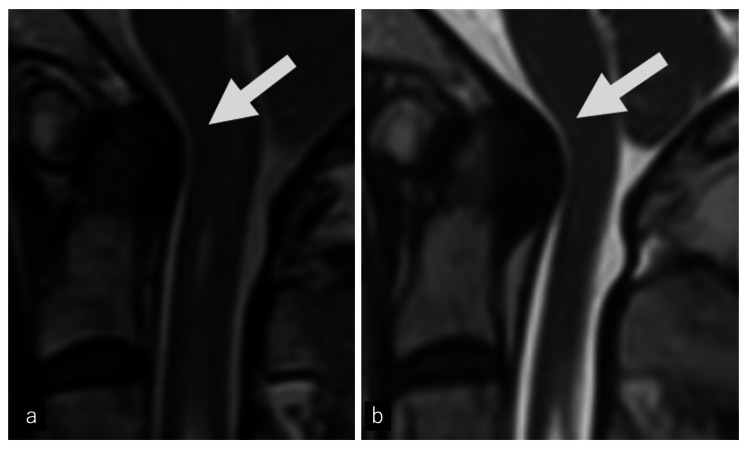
Serial cervical spine magnetic resonance imaging (MRI) 21 years before presentation and at the current presentation (a) Sagittal T2-weighted image 21 years before presentation, (b) Sagittal T2-weighted image at the current presentation. The arrow indicates a retro-odontoid pseudotumor, seen as a newly appeared T2-hypointense mass posterior to the odontoid process on comparison of the current MRI with that obtained 21 years before.
